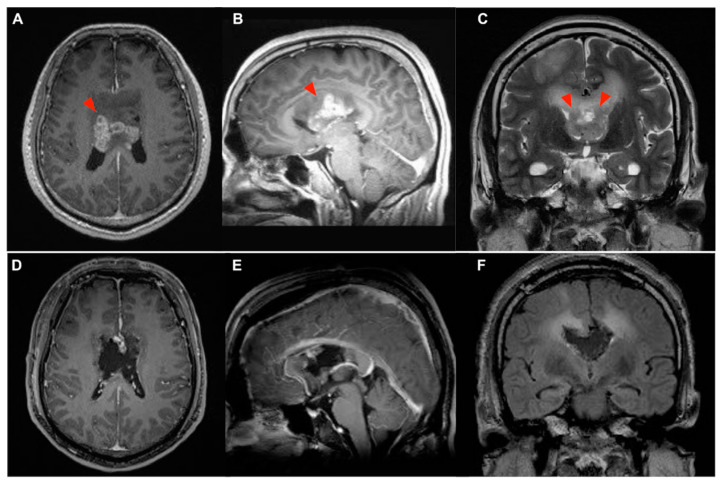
Pre- and postoperative brain MRIs. A large butterfly glioma arising from body of corpus callosum (CC) with heterogeneous enhancement ((A–C), red arrowheads) and T2 high signal extended bilaterally through interhemispheric fibers are shown. A separate non-enhanced lesion at right superior frontal gyrus was shown (C). Enlarged bilateral temporal horns (C) indicated an obstructive hydrocephalus induced by a bilateral occlusion of Foramen of Monro. The postoperative MRI showed a complete removal of contrast enhancing CC tumor (D–F) with a residual T2 high-signal area (F) and resolution of obstructive hydrocephalus (F).
